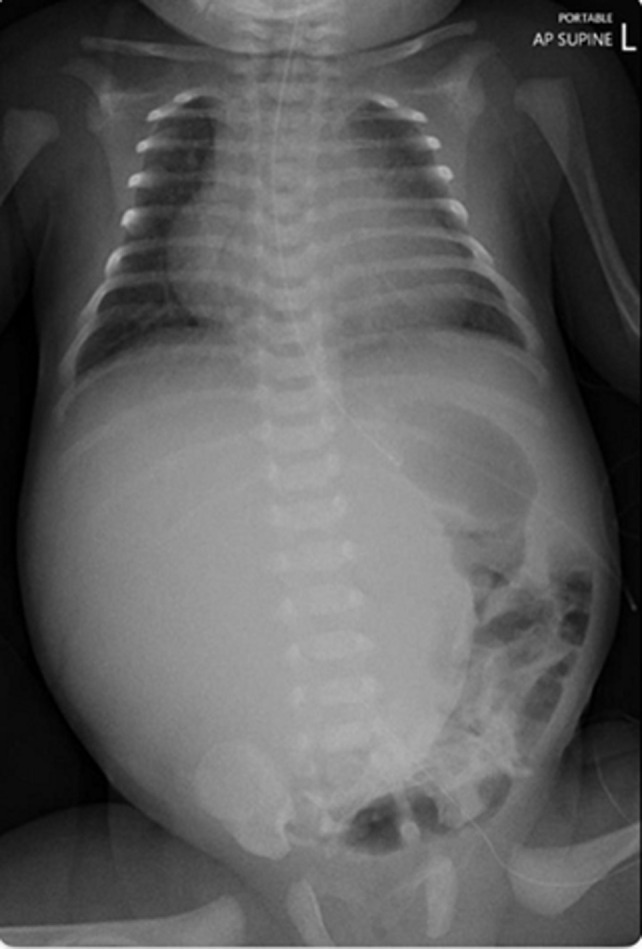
chest and abdomen X-ray showing cardiomegaly and huge hepatomegaly displacing stomach and bowel loops towards the left side
